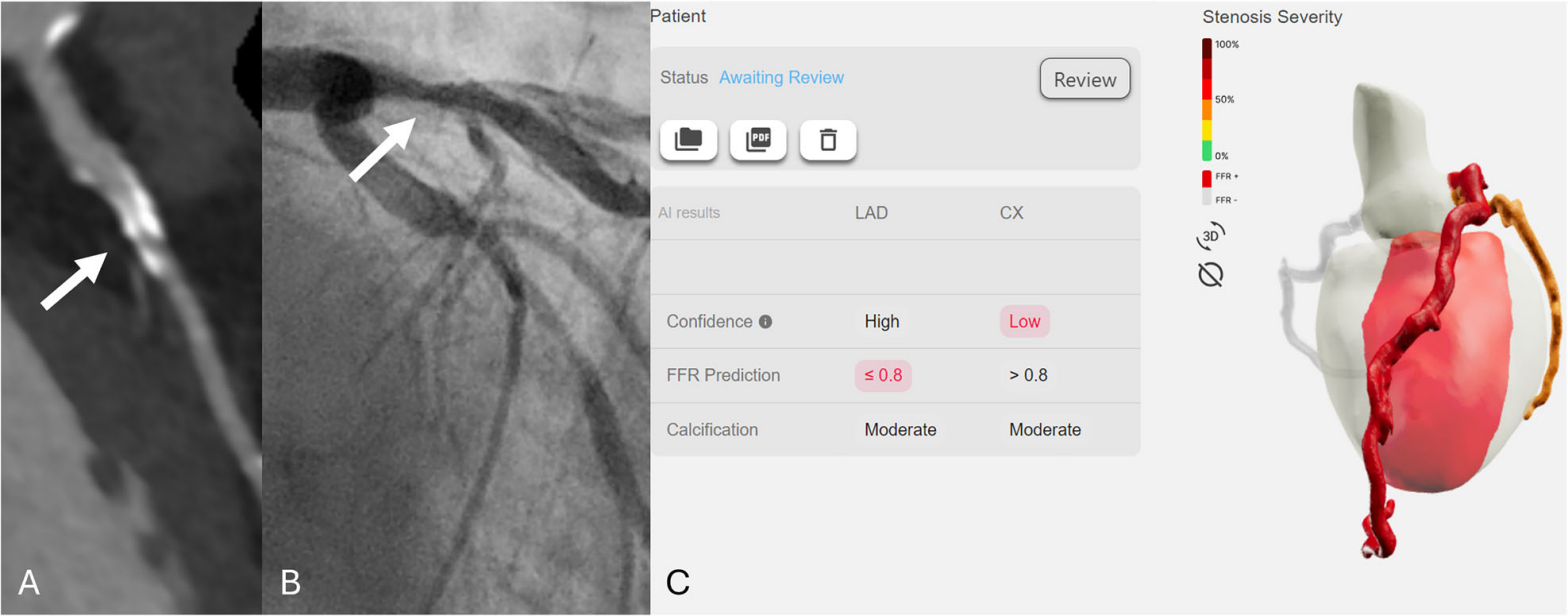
False-positive case. A representative case of an 81-year-old male patient with a recent onset of stable chest pain. Curved multiplanar reconstruction coronary computed tomography angiography A shows a heavily calcified plaque with blooming artifacts (white arrow) in the proximal left anterior descending artery with obstructive stenosis. Invasive coronary angiography B demonstrates a 26–50% stenosis, and Fractional Flow Reserve was 0.89, in contrast to the report of CT-FFR_AI_ that predicts FFR ≤ 0.8 (C). Of note, the patient also had an obstructive stenosis on the left circumflex artery with CT-FFR_AI_ > 0.8 prediction and invasive Fractional Flow Reserve > 0.8 (FFR = 0.91)
